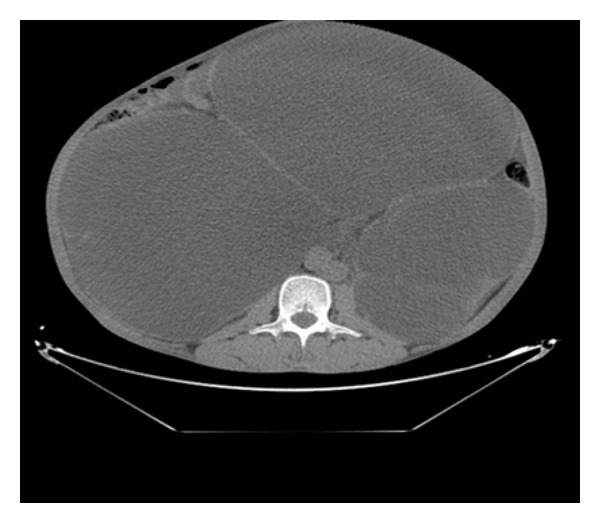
Abdominal CT scan showing massively enlarged bilateral kidney with pressure effect over the bowels.
